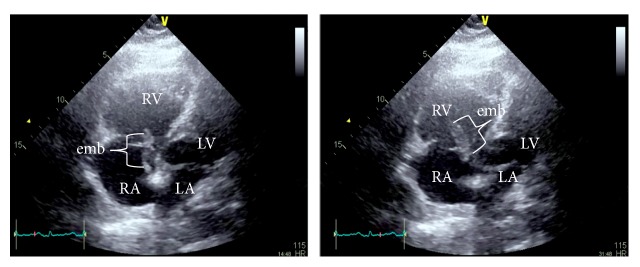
Transthoracic echocardiogram, apical 4-chamber view, showing RV pressure overload with enlarged RV diameter (>41 mm) and a long echodensity (embolus) attached to TV extending in both RA and RV (RHTVE); LA: left atrium; RV: right ventricle; RA: right atrium; LV: left ventricle; TV: tricuspid valve; emb: embolus; RHTVE: Right Heart Transvalvular Embolus.
